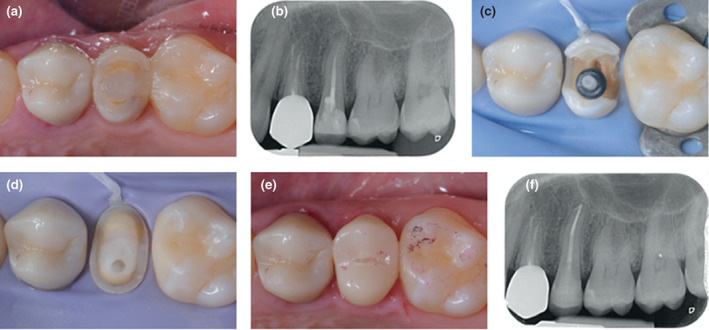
Lithium disilicate onlay (IPS e.max CAD) placement following root canal retreatment of 25 (a, b) preoperative occlusal view and long‐cone periapical radiograph (c) fibre post prior to cementation and core placement (d) completed core and onlay preparation with distal deep margin elevation (e, f) final CAD‐CAM restoration with postoperative occlusal view and follow‐up periapical radiograph.
